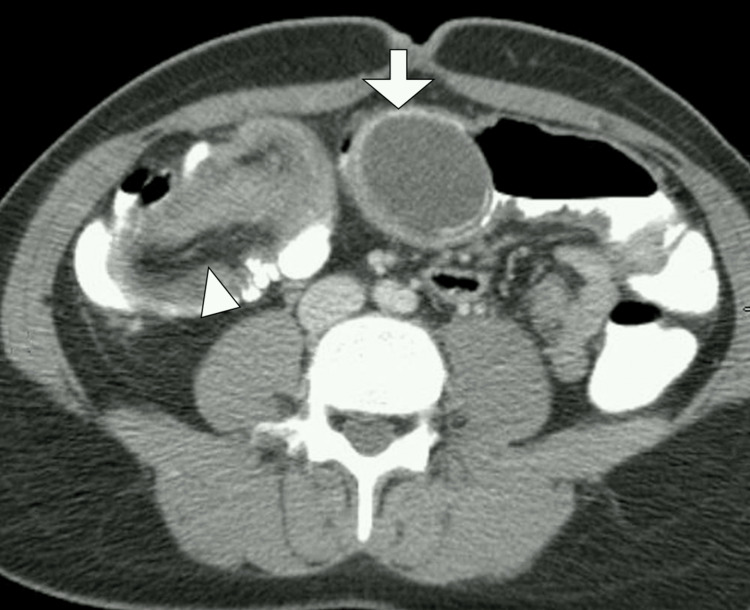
Axial contrast-enhanced CT image of the abdomen and pelvis A distended, fluid-filled appendix consistent with an appendiceal mucocele (arrow) is seen acting as a lead point for ileocolic intussusception (arrowhead) CT: computed tomography
